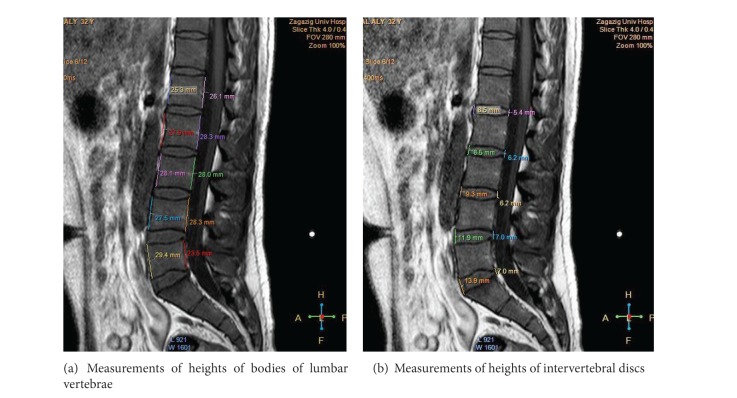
Sagittal T1-weighted MRI of a male aged 32 years showing measurements of lumbar spine segments.
